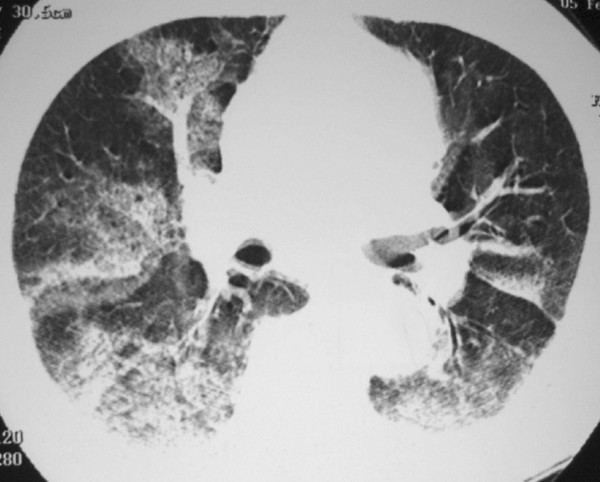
HRCT showing extensive areas of consolidation with air bronchogram and ground glass opacities in both lungs, predominantly in the right lung.
